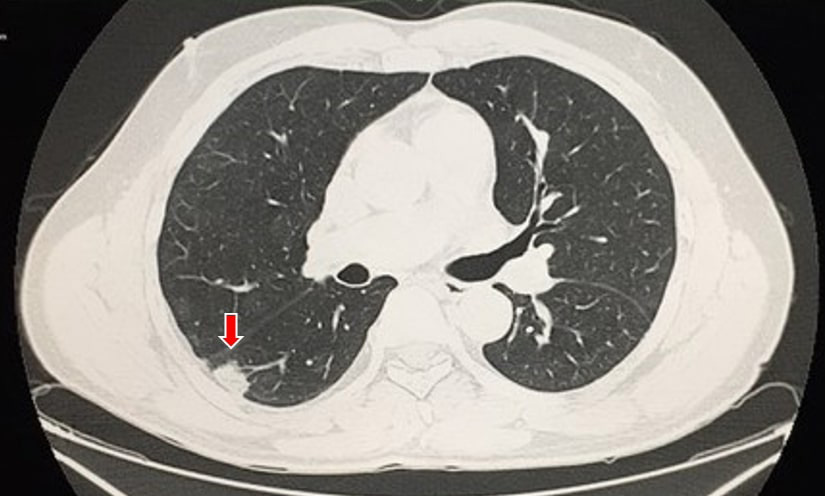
Computed tomography angiography of the chest, with pulmonary parenchyma window, showing area of pulmonary infarction on the right (arrow).
